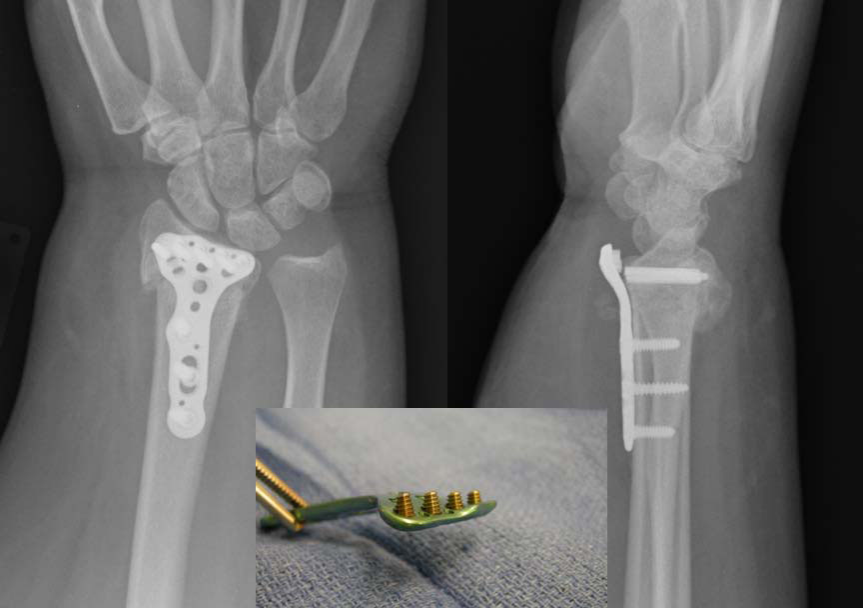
Radiographs show collapse of the fracture following failure of screws. Despite the collapse, the lateral radiograph showed neutral alignment of the distal radius articular surface with minimal shortening on the posteroanterior (PA) plane.
